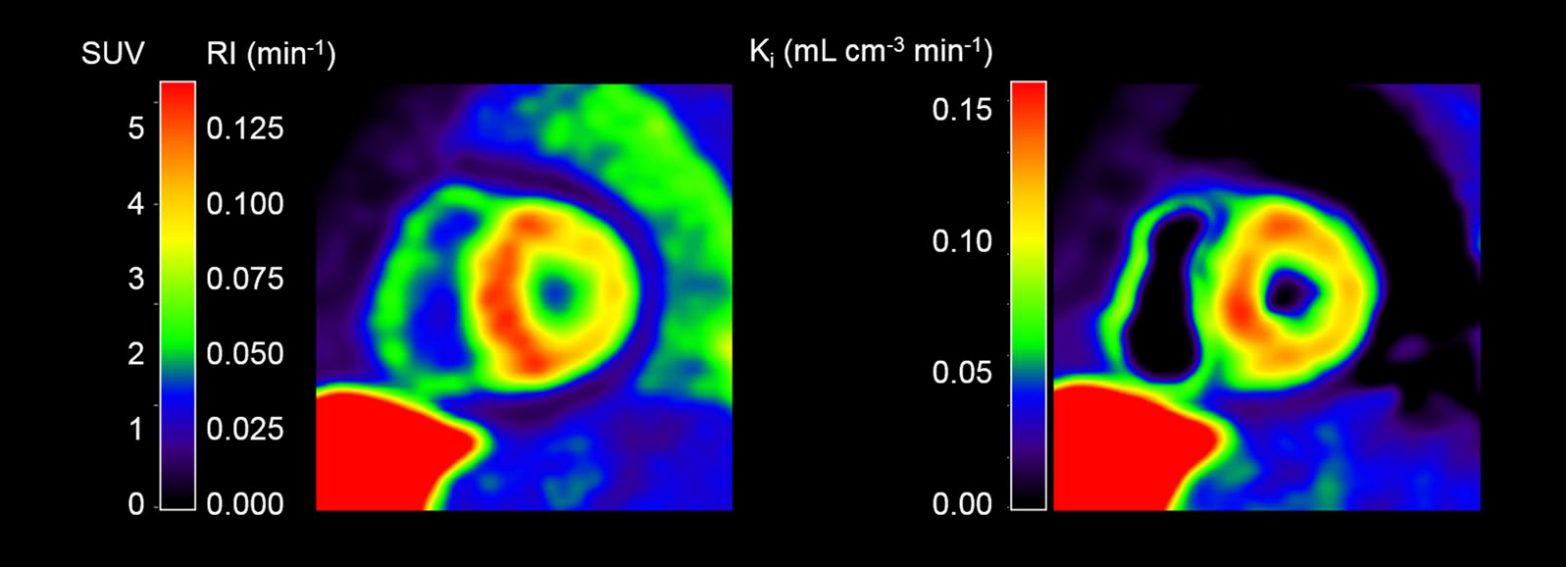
Cardiac short axis ^11^C-PIB images from a patient with AL-amyloidosis. Left: SUV/RI image. Right: Net influx rate *K*_i_ image calculated using a basis function implementation of the 2Tirr model
